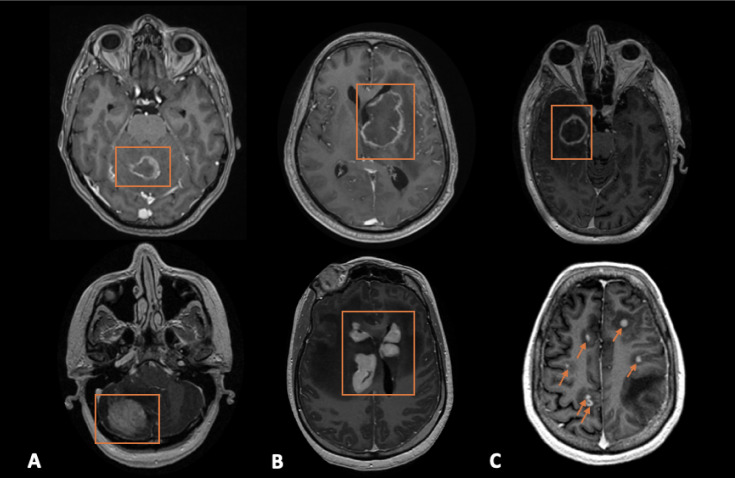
Examples of lesion distribution on contrast-enhanced T1-weighted sequences. A Infratentorial location, upper row: necrotic lesions, lower row: homogeneous lesions; B Deep location (basal ganglia and periventricular region), upper row: necrotic lesions, lower row: predominantly homogeneous lesions; C Peripheral location, upper row: necrotic lesions, lower row: predominantly homogeneous lesions
